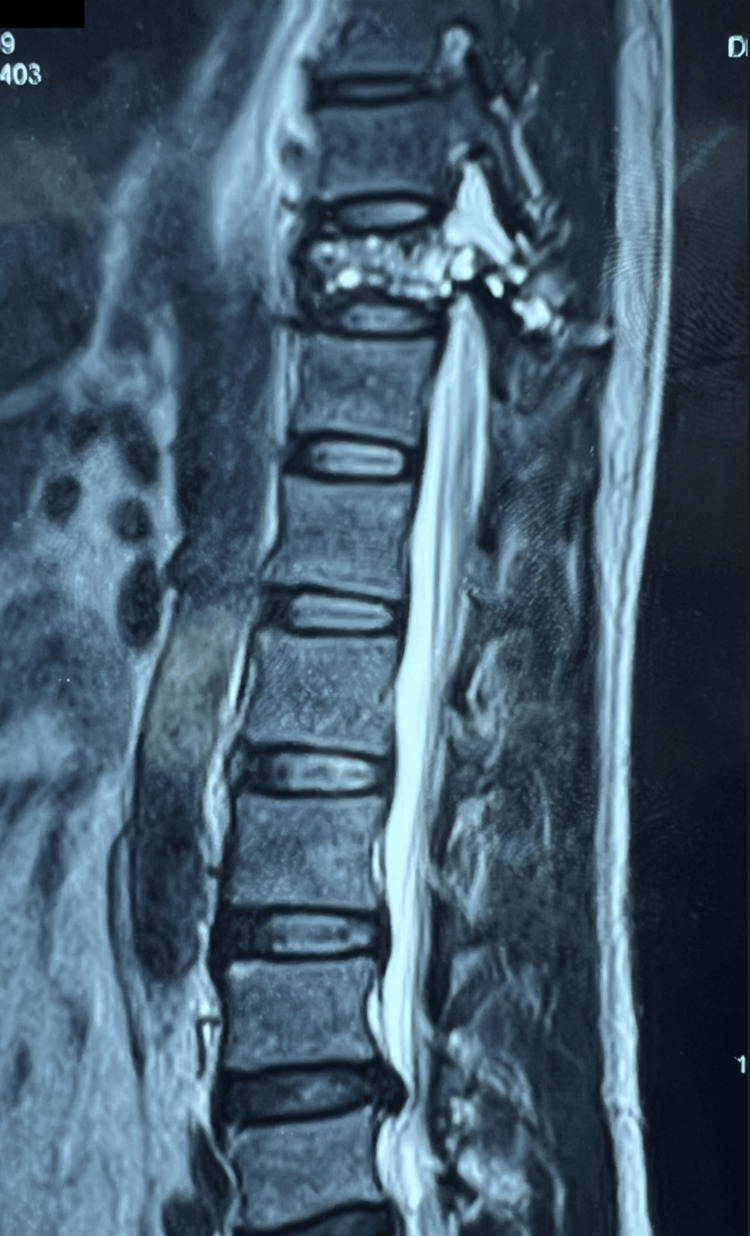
MRI scan of thoracolumbar spine with contrast, T2-weighted (sagittal section) showing characteristic findings of aneurysmal bone cyst: multiloculated lesion at T11 vertebra with multiple fluid-fluid levels.
